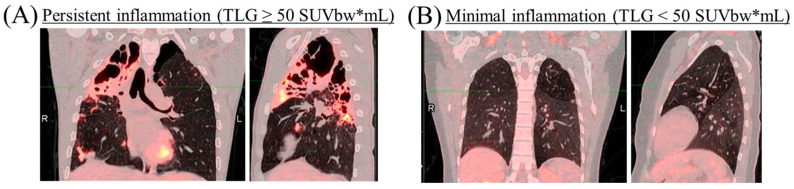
Representative PET/CT images of participants who successfully completed a 6-month drug-sensitive anti-TB treatment, showing the lung in coronal view (left) and lateral view (right). (A) Participant with extensive persistent lung inflammation (TLG ≥ 50 SUVbw*mL) and (B) Participant with minimal lung inflammation (TLG < 50 SUVbw*mL).
